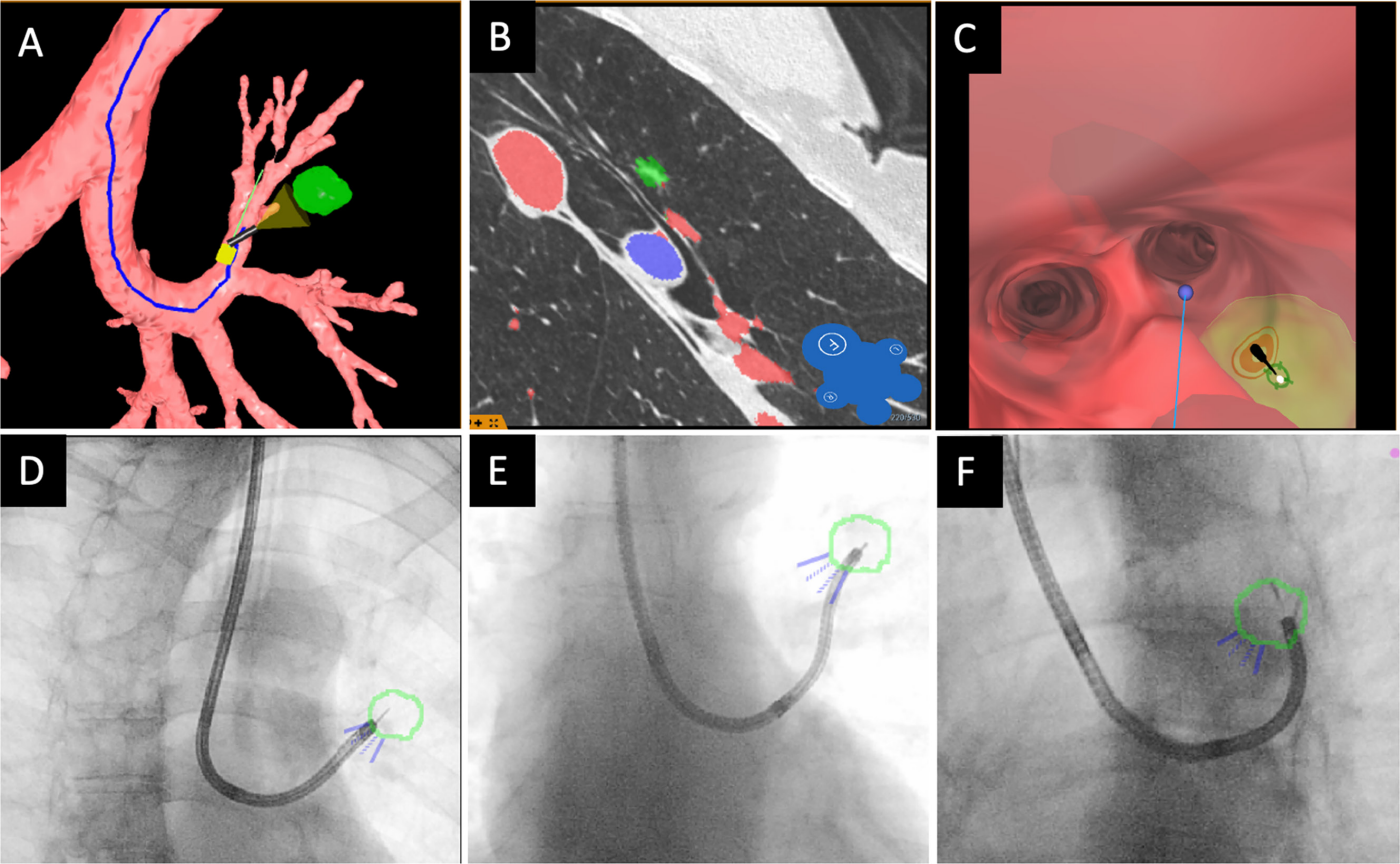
Virtual navigation planning (A–C) and intraoperative real‐Time biopsy guidance with fused fluoroscopy (D–F). A: View of the virtual 3D reconstruction with the marked navigation path (blue line) leading to the target lesion in the left upper lobe (green area). B: Annotation of vascular structures enables the activation of the virtual Doppler function (C) within the endoscopic virtual navigation view. The target lesion is visible as a greenish area, with the red target marker centrally indicating the point of entry for tunnel creation. The reddish structures visible through the bronchial mucosa represent vascular structures. D–F: Fused fluoroscopy with the target lesion (green segmentation) derived from the pre‐procedural CT scan and VBN segmentation. Utilising a C‐arm, the biopsy tool is positioned within the virtual target in three different planes, indicating successful virtual target attainment.
